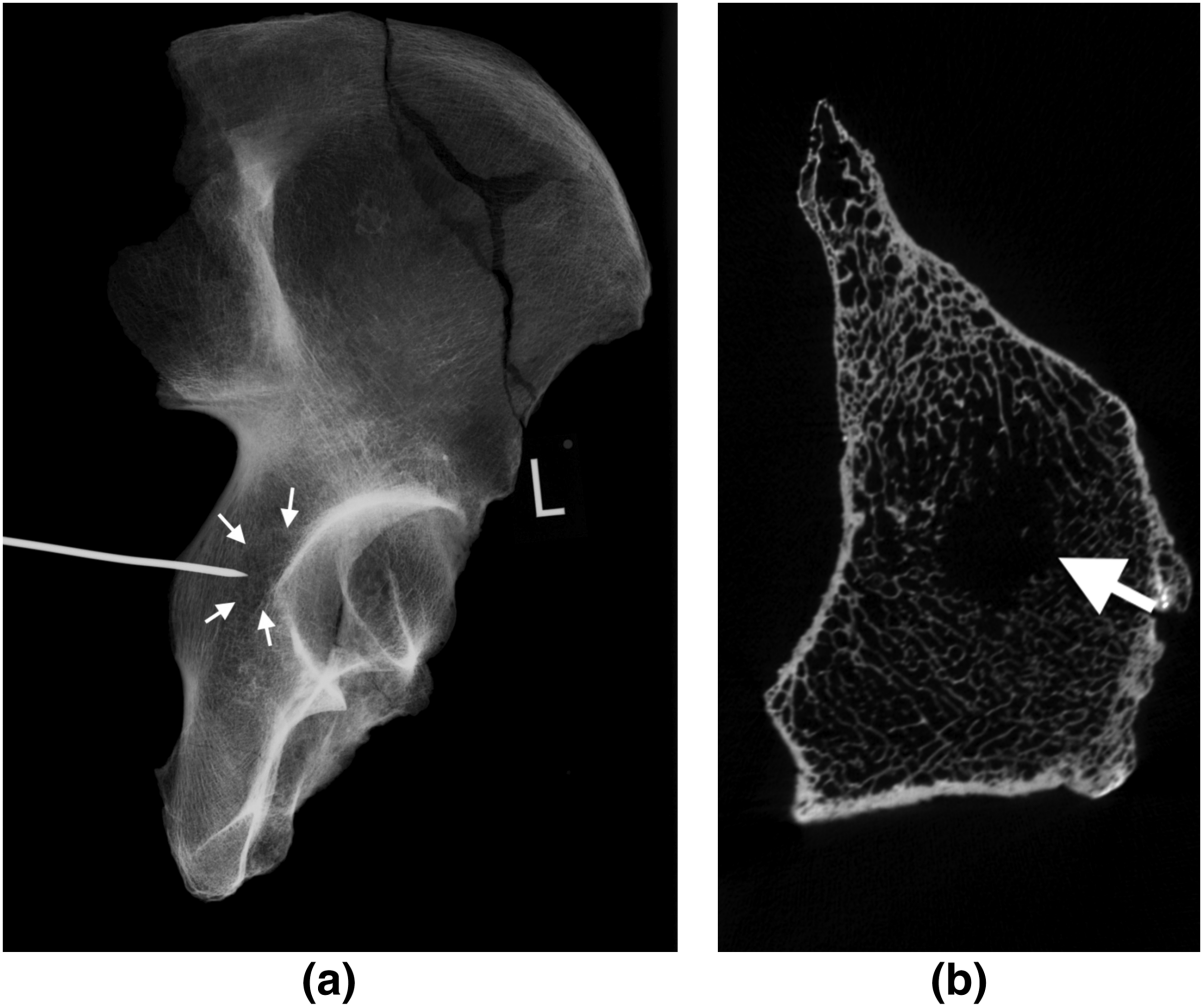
(a) Plain radiograph with the tip of metal pointer located over a lytic metastasis (highlighted with arrows) in left hemipelvis of PSN737, close to the acetabulum; (b) CT shows the metastasis more clearly, located in the cancellous bone (arrow). Image credit: Bram Mulder
